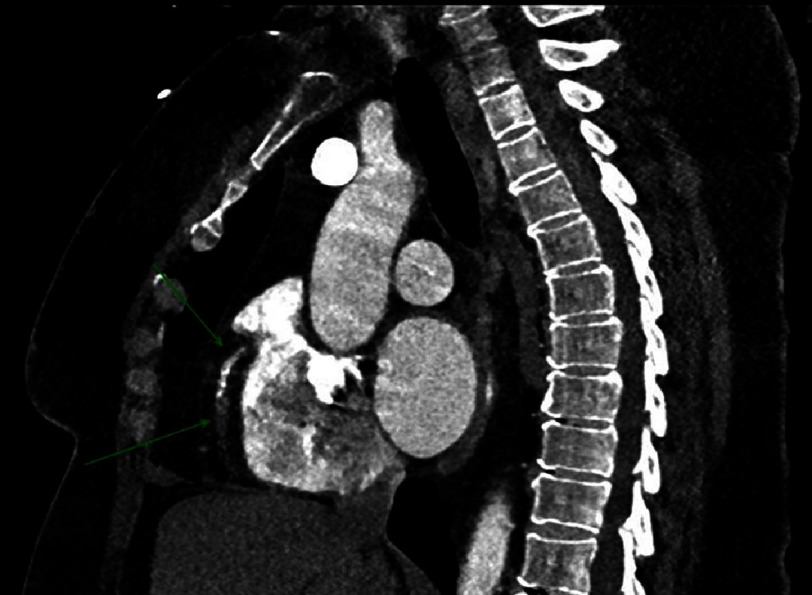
The CT chest with arterial contrast timed for dissection evaluation. The green arrows point to the RCA that appears to be occluded.
